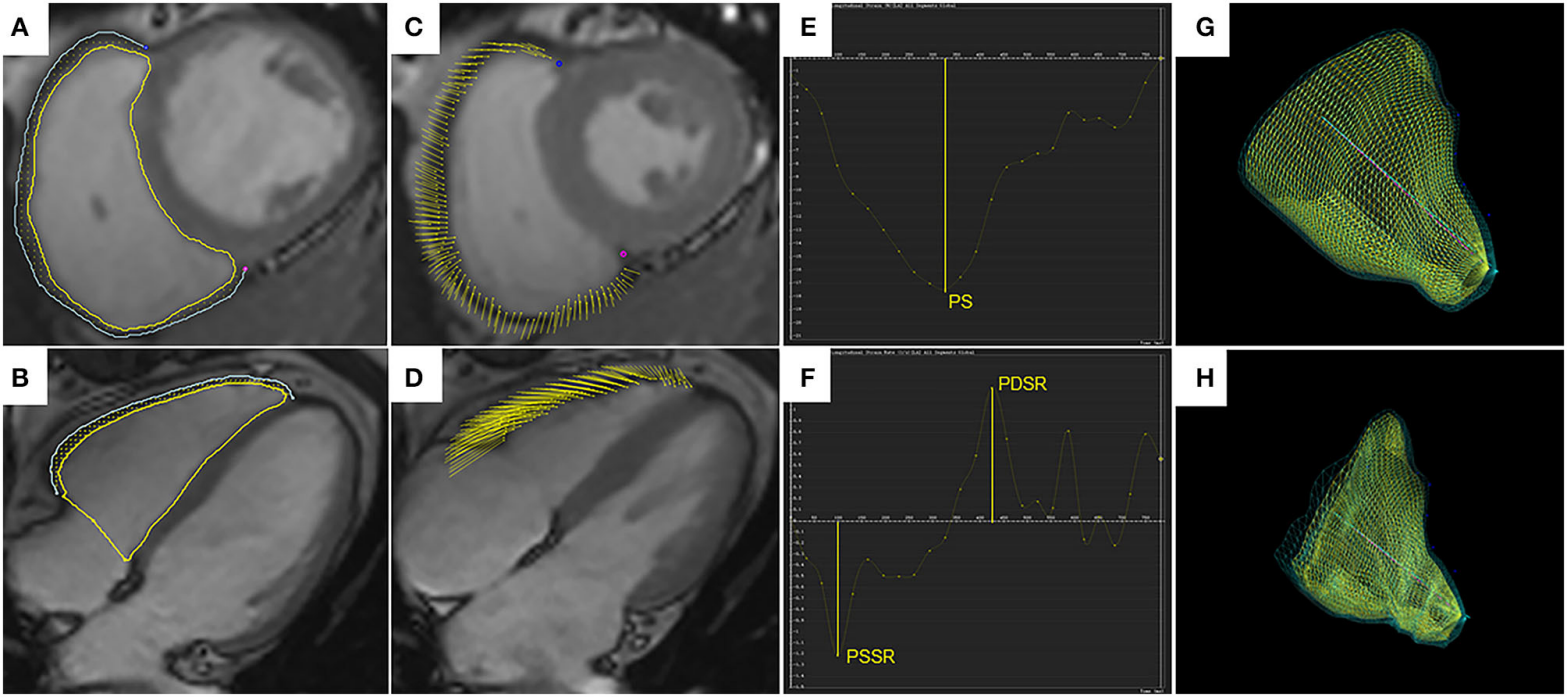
Right ventricular tissue tracking using cardiac magnetic resonance imaging. Right ventricular (RV) contours are delineated on the short-axis SSFP image (A) and the four-chamber image (B), shown for the end-diastolic phase. Yellow and blue lines represent RV endocardial and epicardial borders, and yellow dots between the borders represent myocardial points. (C,D) demonstrate myocardial points motion from the end-diastolic phase to the end-systolic phase using tissue tracking. (E,F) show strain curve and strain rate curve. The peak strain (PS) and strain rates (PSSR and PDSR) can be acquired in the curves. 3D models of the RV in the end-diastolic phase (G) and the end-systolic phase (H). SSFP, steady-state free precession; PS, peak strain; PSSR, peak systolic strain rate; PDSR, peak diastolic strain rate; 3D, three-dimensional.
